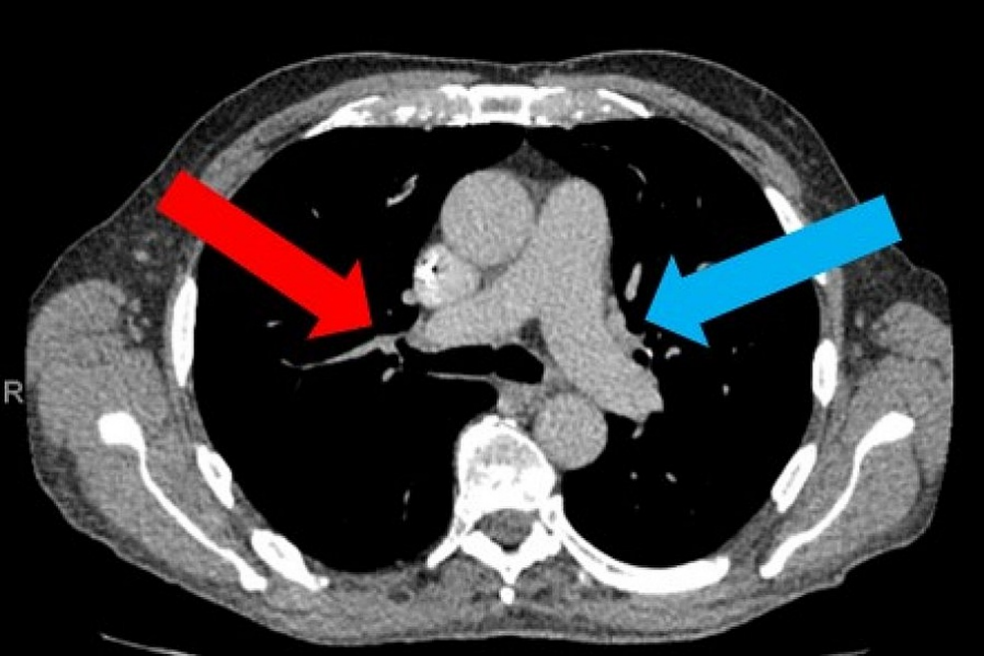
CT chest showing enlarged sub-aortic lymph nodes (blue arrow) and right lower paratracheal nodes (red arrow)
